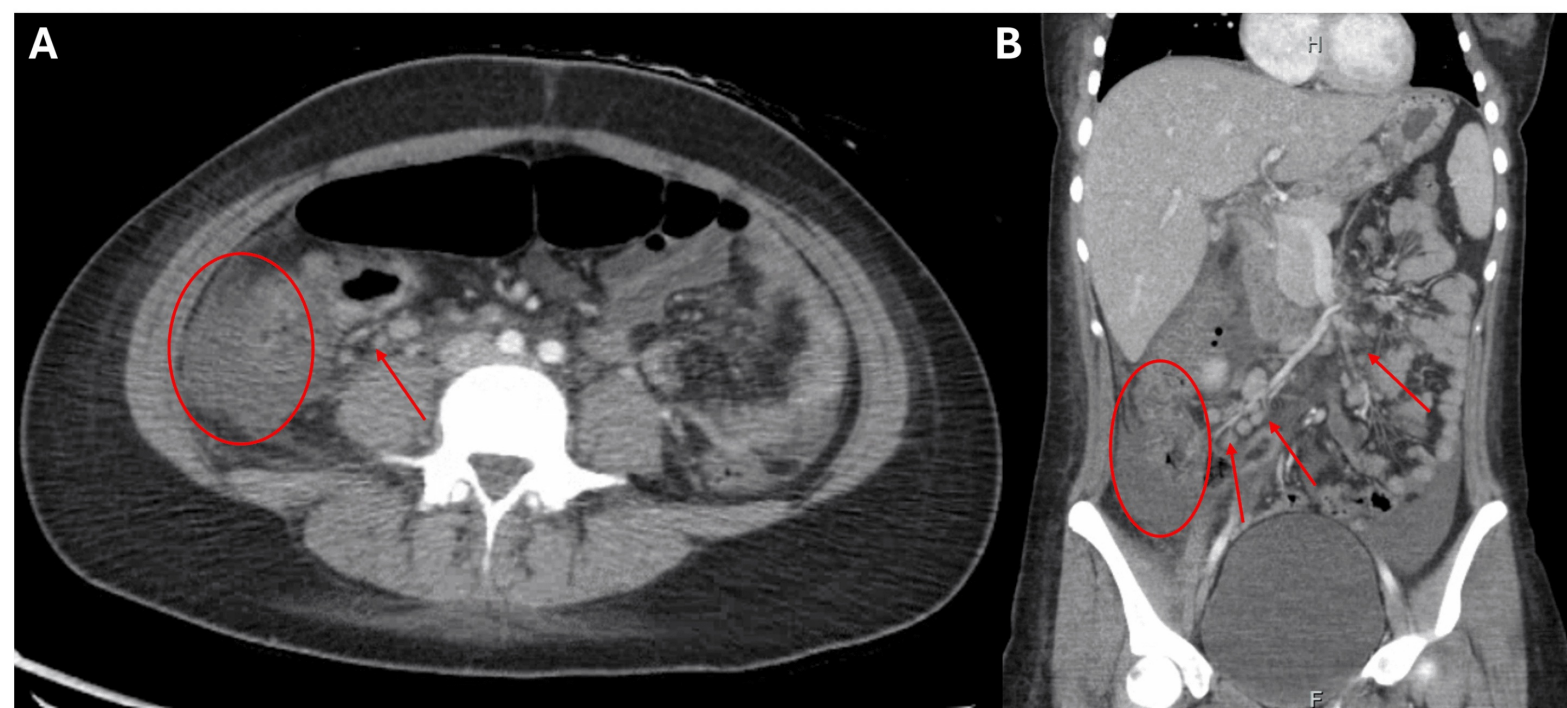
Patient’s CT imaging of the abdomen and pelvis with contrast (A) Transverse section of the patient’s abdomen. (B) Coronal section of the patient’s abdomen. There was nonspecific right-sided colitis with ascending colon wall thickening, hyperemia, and adjacent fat stranding (ovals). There was also diffuse mesenteric adenitis with mildly enlarged lymph nodes, most prominently in the right lower quadrant (arrows).
